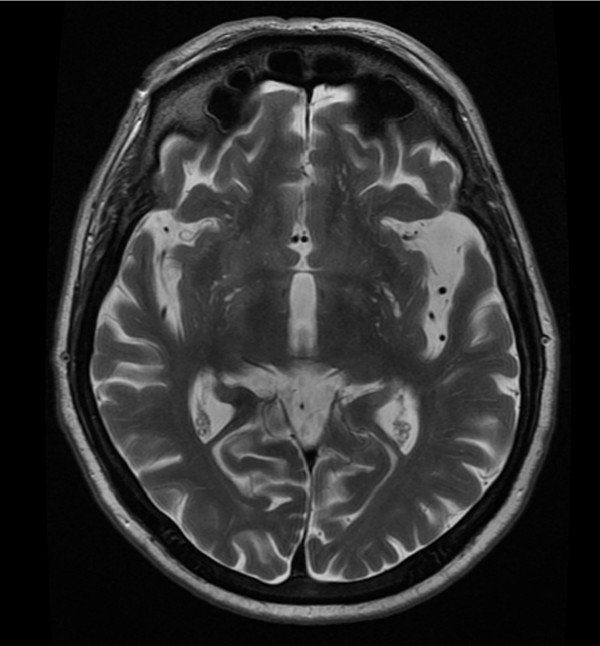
Magnetic resonance imaging. Axial T2-weighted sequence showing diffuse cortical atrophy predominating at the left sylvian fissure.
